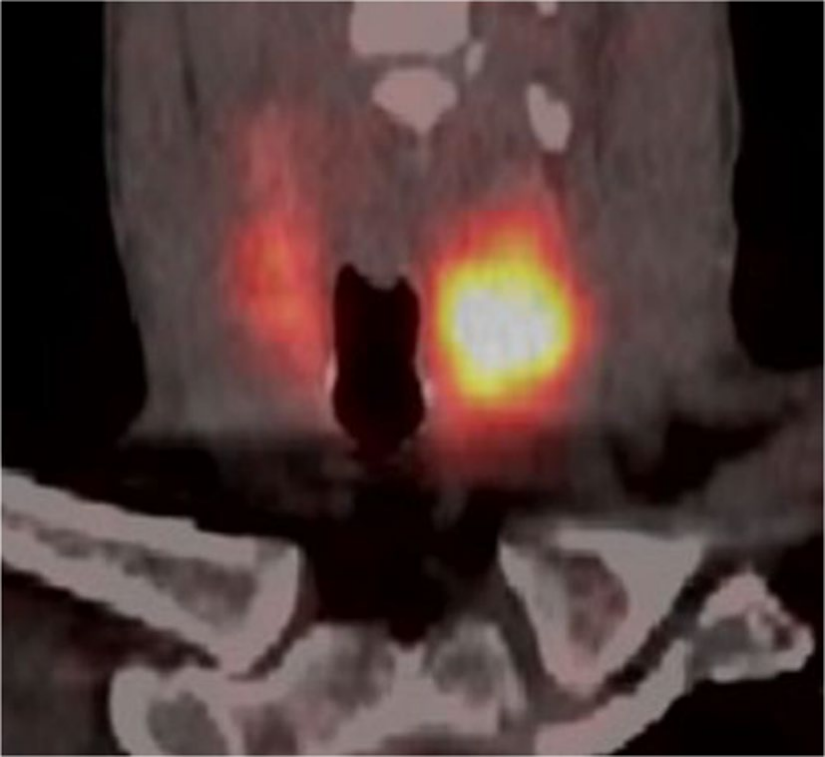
^68^Ga-DOTATATE PET/CT coronal fusion image showing high uptake in the left thyroid nodule and mild and diffuse physiological uptake in the right lobe
